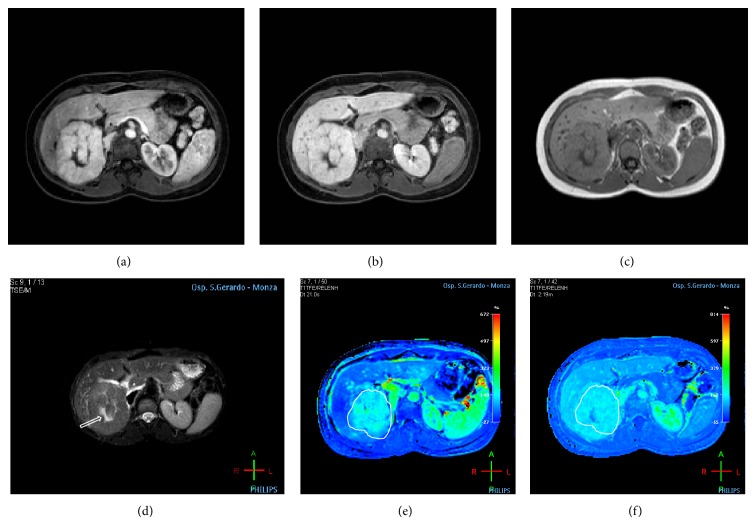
A 32-year-old woman with abdominal pain. The MR imaging shows a focal lesion in the right lobe. (a, b) Axial arterial and delayed postcontrast injection images; the lesion appears as a well-shaped hyperintense area in the arterial phase, with isointensity signal in delayed phase, with presence of hypointense central scar (arrow). (c) Axial T1-weighted in-phase echo-gradient sequence shows isointense irregular nodular area in the sixth segment. (d) Axial T2 fat-sat weighted sequence shows a rounded ill-defined nodular area, with slight intensity signal on T2-weighted images and with hyperintense scar (arrow) on the central part. (e, f) On colour perfusion maps (Relative Arterial Enhancement and Relative Late Enhancement, resp.) the lesion (ROI contouring the boundaries of the lesion) corresponds to hypervascular area.
